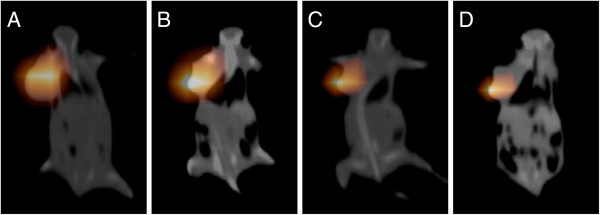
The results of SPECT imaging at 1 (A), 4 (B), 8 (C) and 16 days (D) after intratumoral administration of ^131^I-GMSs. The injected radioactivity concentrated in the tumors, and no accumulation of radioiodine was noted by SPECT scan in other tissues.
